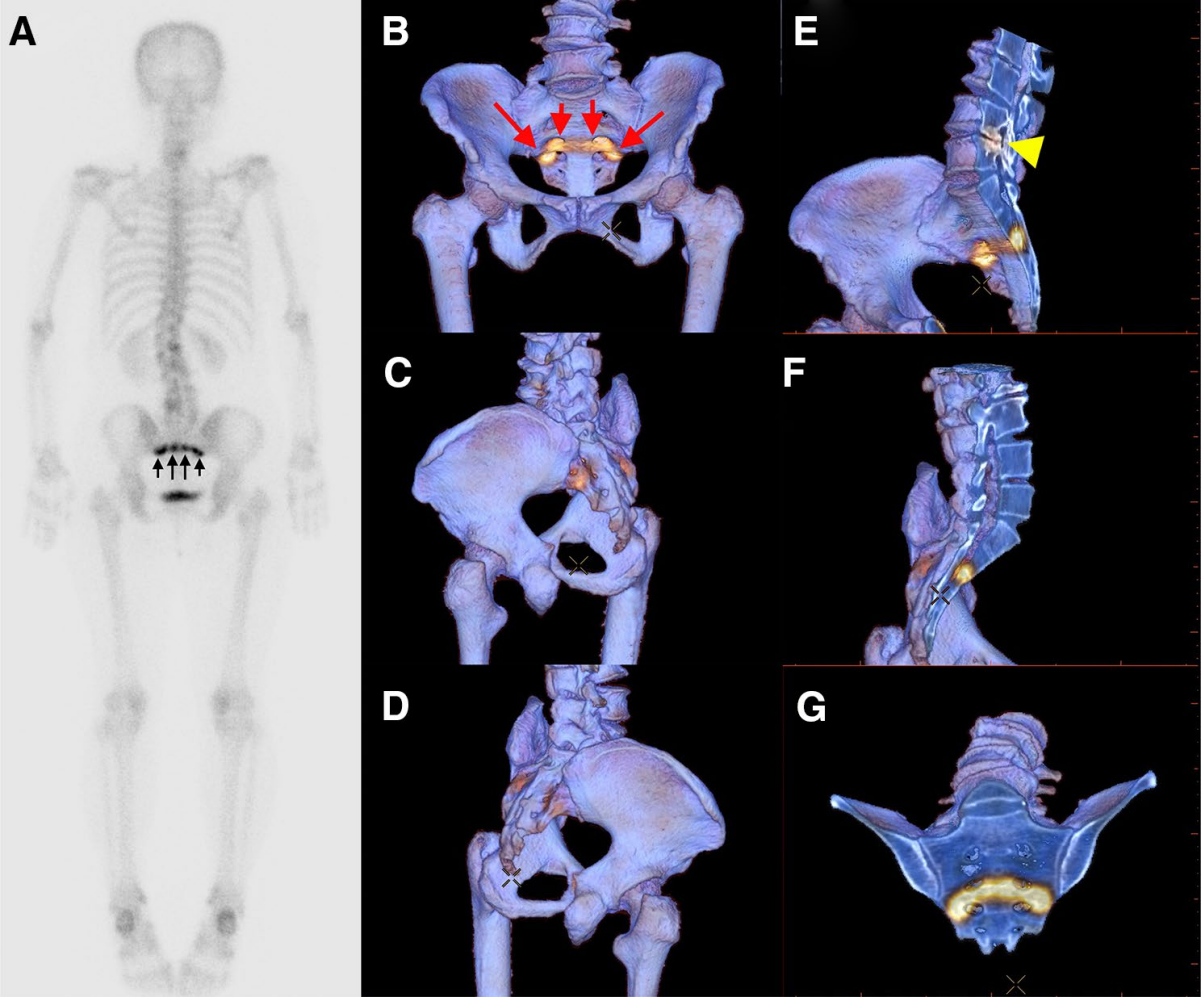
Application of “clip-plane editing” to our data expression of bone SPECT into 3D volume-rendered CT images. Whole-body two-dimensional scintigraphy in a breast cancer patient shows band-like uptake in the sacrum (a, *arrows*). The three-dimensional fused images with our method show that the uptake is mainly observed in the anterior side of the sacrum (b–d, *arrows*). Cross-sectional images of the 3D data show that the uptake is not tumor-like, making the diagnosis of insufficiency sacral fracture (e–g). In addition, osteoarthrosis around the joint between the fourth and fifth lumbar spine with increased metabolic activity is seen (e, *arrowhead*)
